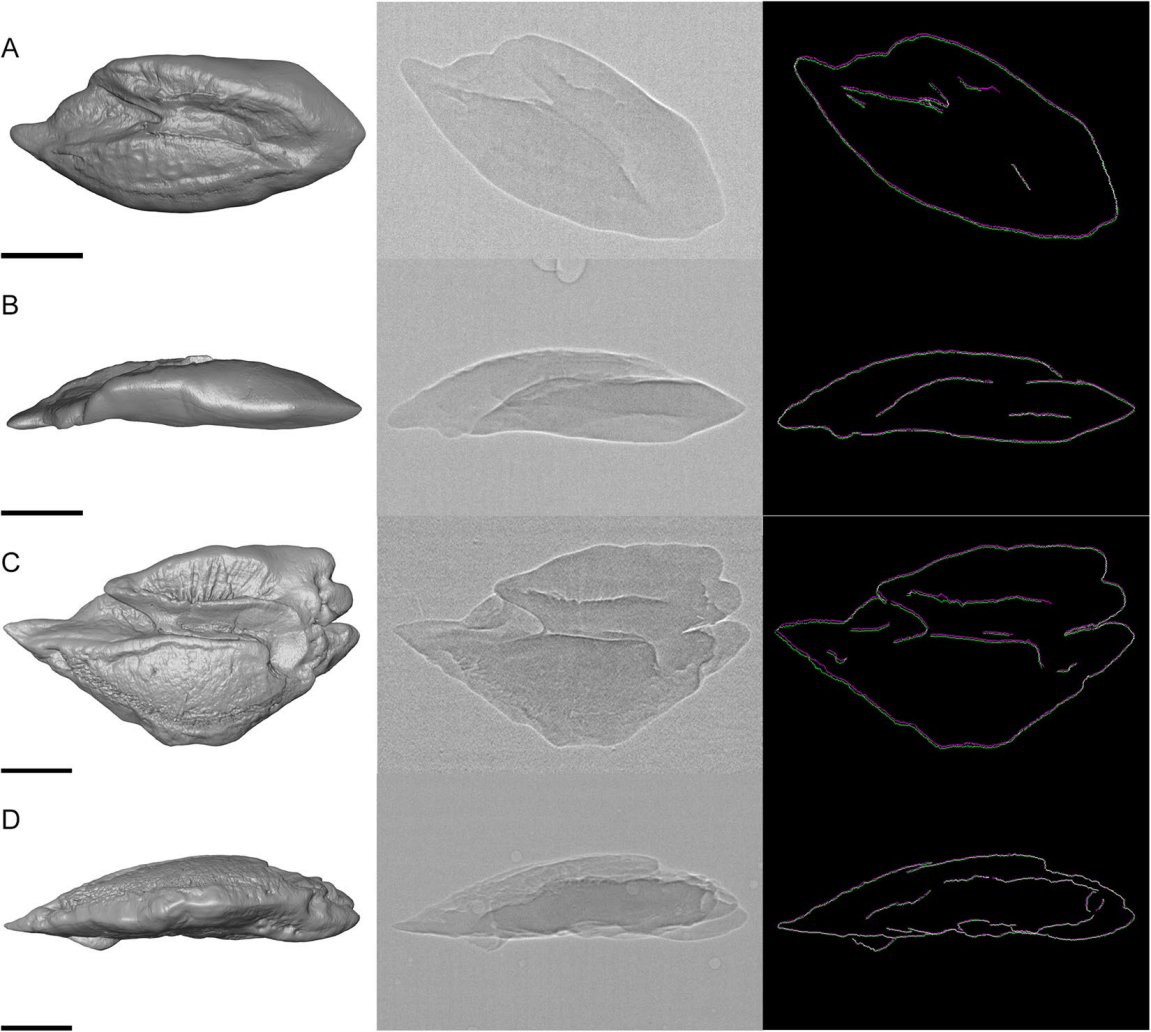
Saccular otoliths of *S*. *tinanti* (A,B) and *E*. *maculatus* (C,D) used for the experiments in 1% agarose shown as right otoliths. (A,B) represent mirror images of the left otolith of *S*. *tinanti*. The first column shows 3D reconstructions of the otoliths in medial (A,C), dorsal (B), or ventral (D) views. 2D radiographic images of the same otoliths are shown in the middle column. The right column displays the overlays of averaged maximum (purple) and minimum (green) positions of the otolith during otolith motion due to stimulus presentation. Scale bars, 500 µm.
